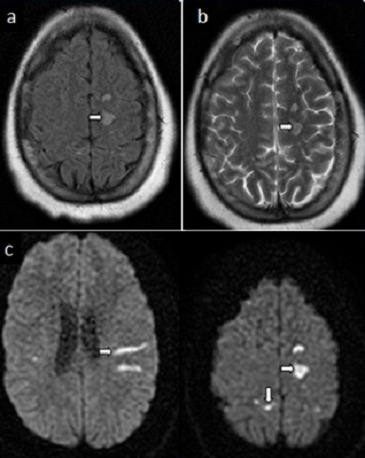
IRM cérébrale en séquence T2(a), FLAIR(b), et diffusion(c) a révélé plusieurs lésions (flèches) en corticales et sous-corticales temporo-fronto-pariétales gauches et temporo-pariétales droites, hyperintenses, punctiformes et linéaires de nature ischémique et de systématisation artérielle
